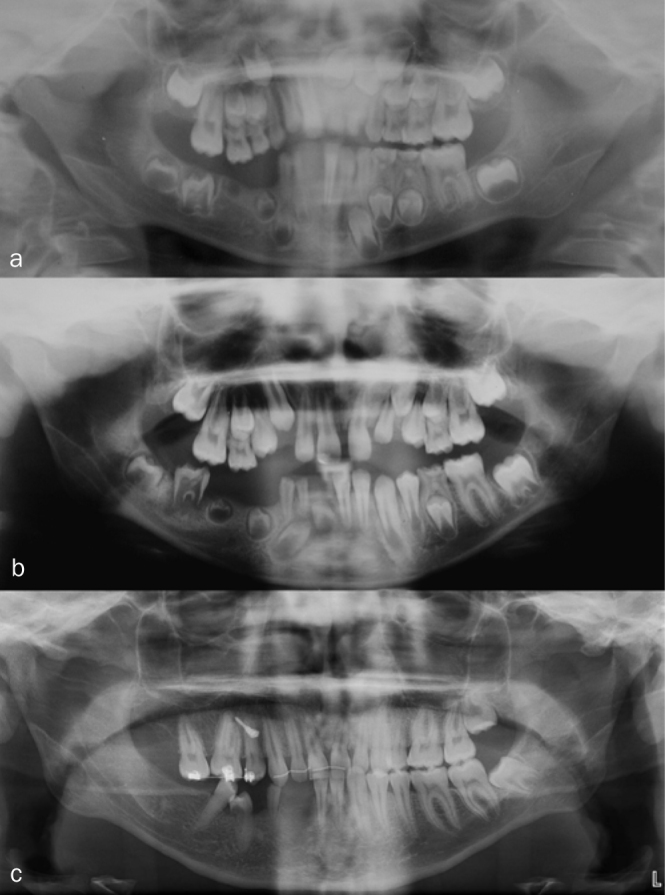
Treatment of patient G, who was followed for 19 years (from 3 to 21 years of age). a) Orthopantomogram at age 8. Teeth 47, 46, 45, 44, 43, 42, and 41 are affected by RO. Note the supraposition of teeth in the maxillary right quadrant. b) Orthopantomogram at age 10. Tooth 46 is erupting despite minimal root formation. c) Orthopantomogram at age15. Teeth 47, 46, 45, 44, 43 and 42 extracted. Autotransplantation of 15 and 25 to region 45 and 44. Note the radiolucency on ‘44’, this tooth was latter lost due to ankylosis.
